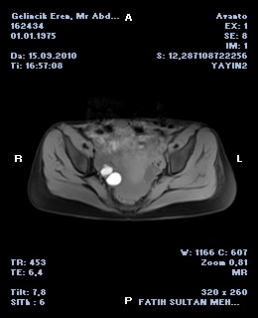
Hemorrhagical fluid was detected in MRI.
